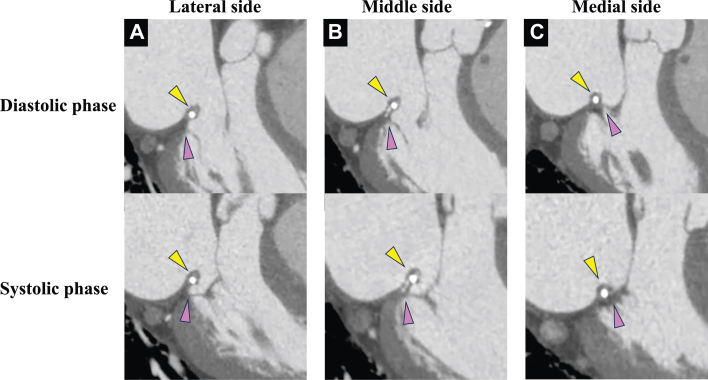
Computed Tomography Findings Lateral side (A), middle side (B) and medial side (C) of mitral valve are shown. Yellow arrowheads indicated prosthetic valve ring and pink arrowheads indicated the original mitral valve annulus.
